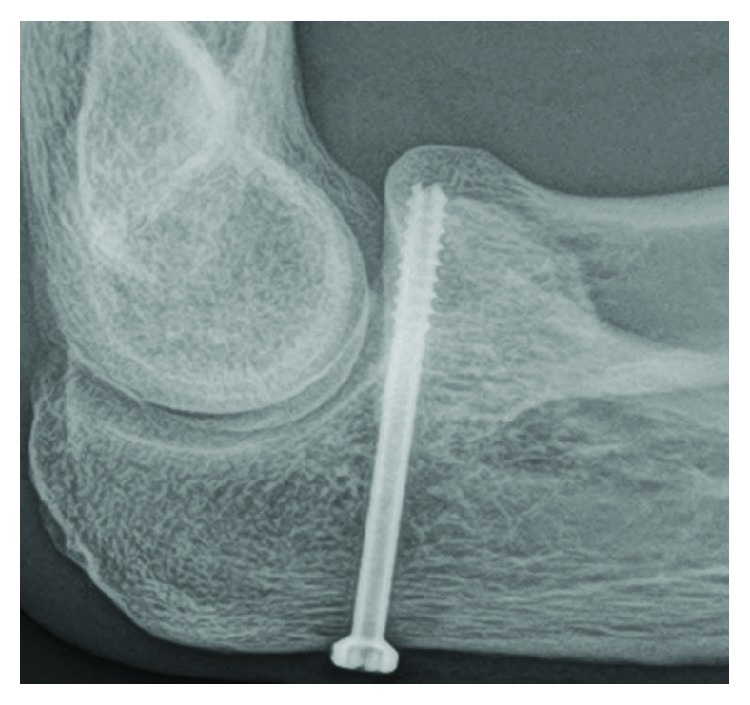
Case 1: radiograph 4 months after the operation shows bone union.
